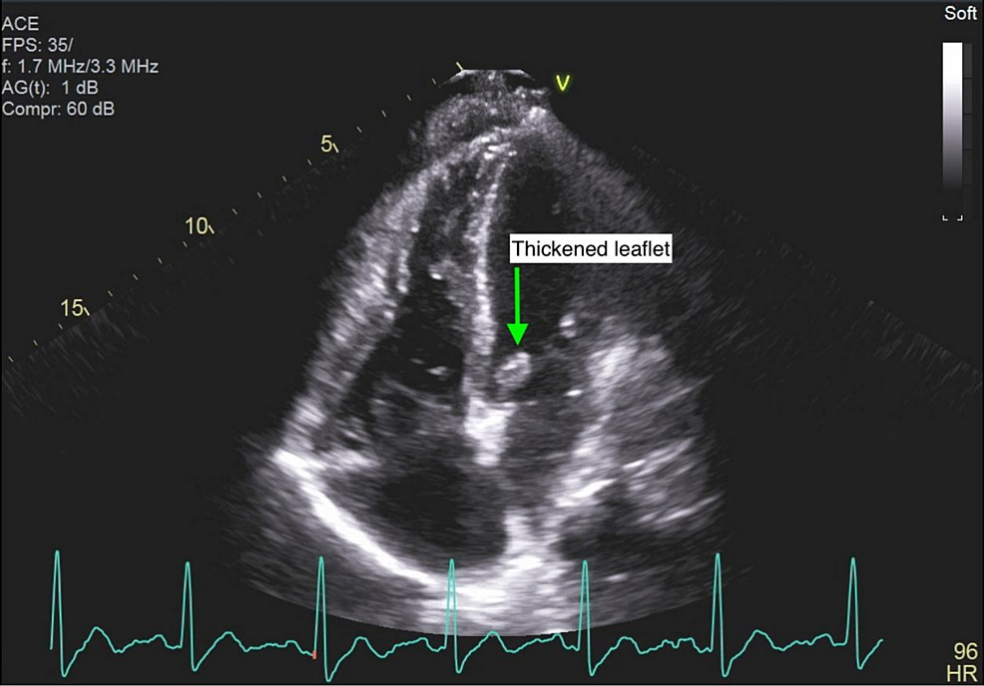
Transthoracic echocardiogram showing thickening of the posterior leaflet subvalvular apparatus of the mitral valve.
